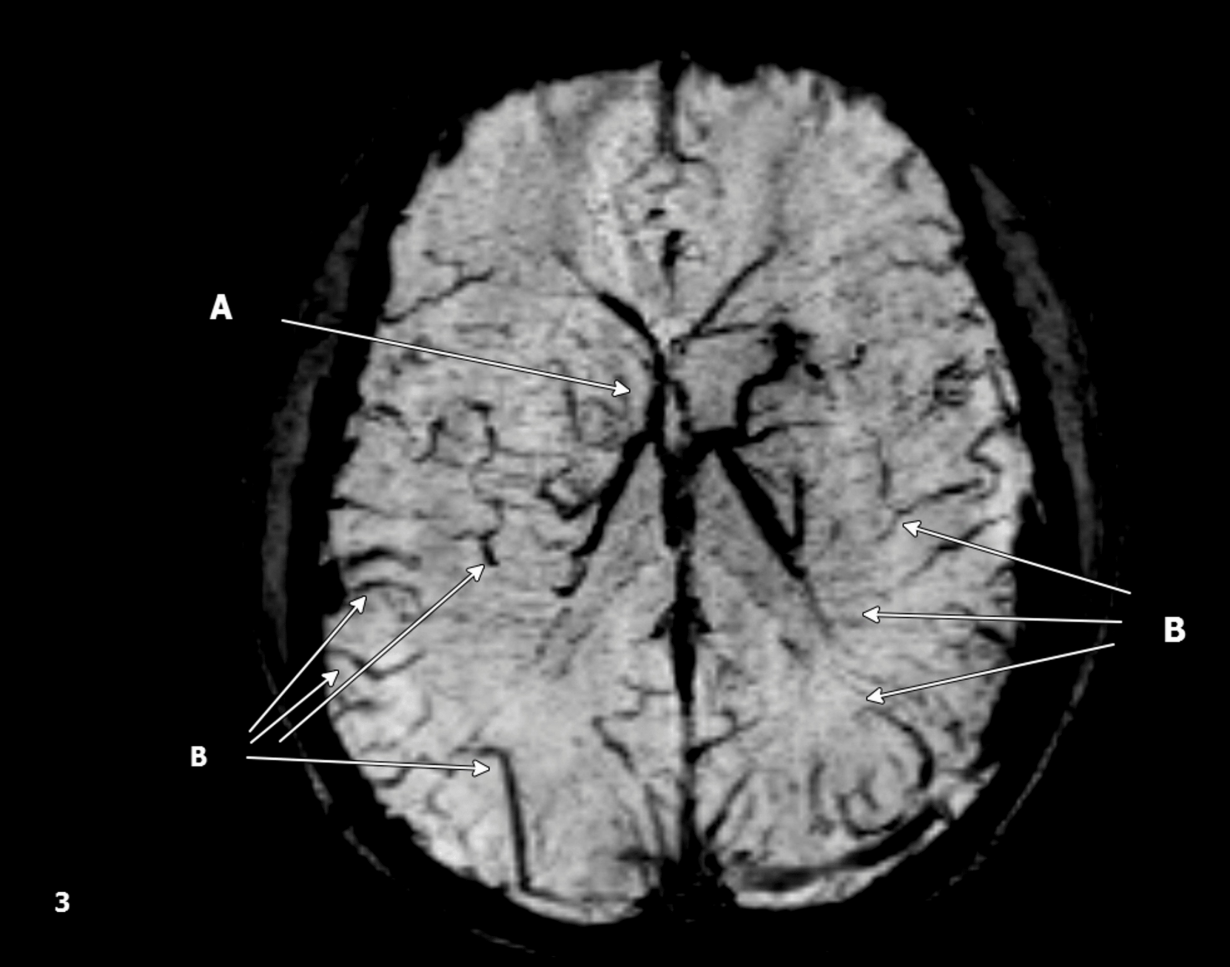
Axial SWI MRI sequence findings suggestive of venous congestion: (A) lateral ventricles with radiating linear hypointense SWI foci and (B) cerebral sulci and white matter with linear SWI signal loss and faint enhancement, likely venous congestion/stasis. SWI: Susceptibility Weighted Imaging, MRI: Magnetic Resonance Imaging
